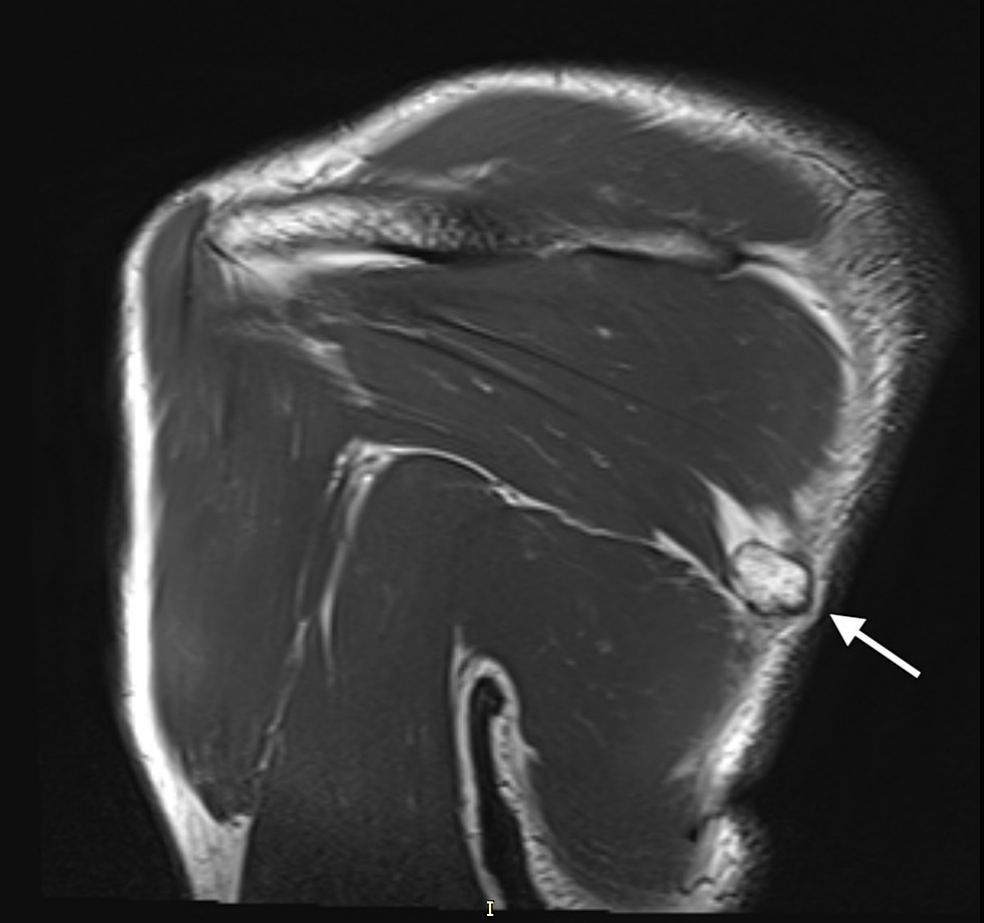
MRI coronal view showing a hyperintense lesion on the T2-weighted image measuring 1.58 × 1.80 × 1.54 cm (AP × TR × CC) at their maximum lengths on the dorsomedial aspect of the left scapula. MRI: magnetic resonance imaging; AP: anteroposterior; TR: transverse; CC: craniocaudal
